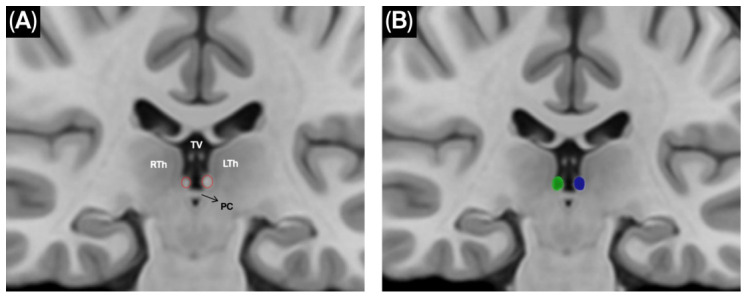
Manual delineation of the bilateral habenulae on the HCP-1065 template. (A) Coronal template view demonstrating the epithalamic region and key anatomical landmarks used to localize the habenulae. The right and left habenulae are highlighted by red dotted outlines. Abbreviations: RTh, right thalamus; LTh, left thalamus; PC, posterior commissure; TV, third ventricle. (B) Corresponding ROI placement for tractography on the same T1-weighted template background. The manually delineated ROIs are shown as the right habenula (green) and the left habenula (blue).
